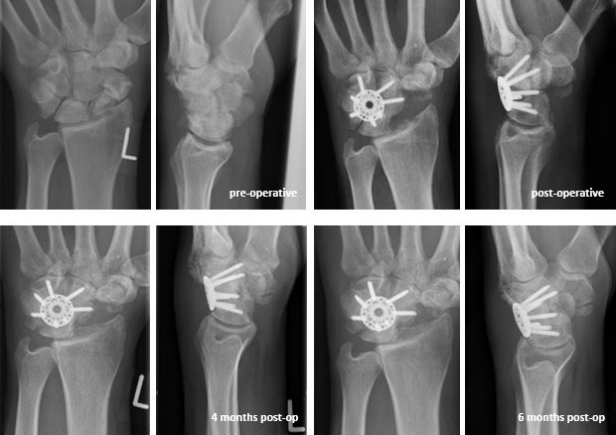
X-ray series of an advanced carpal collapse (SNAC wrist II–III) treated with Synthes^®^ VA LCP ICF Plate up to six months after operation
